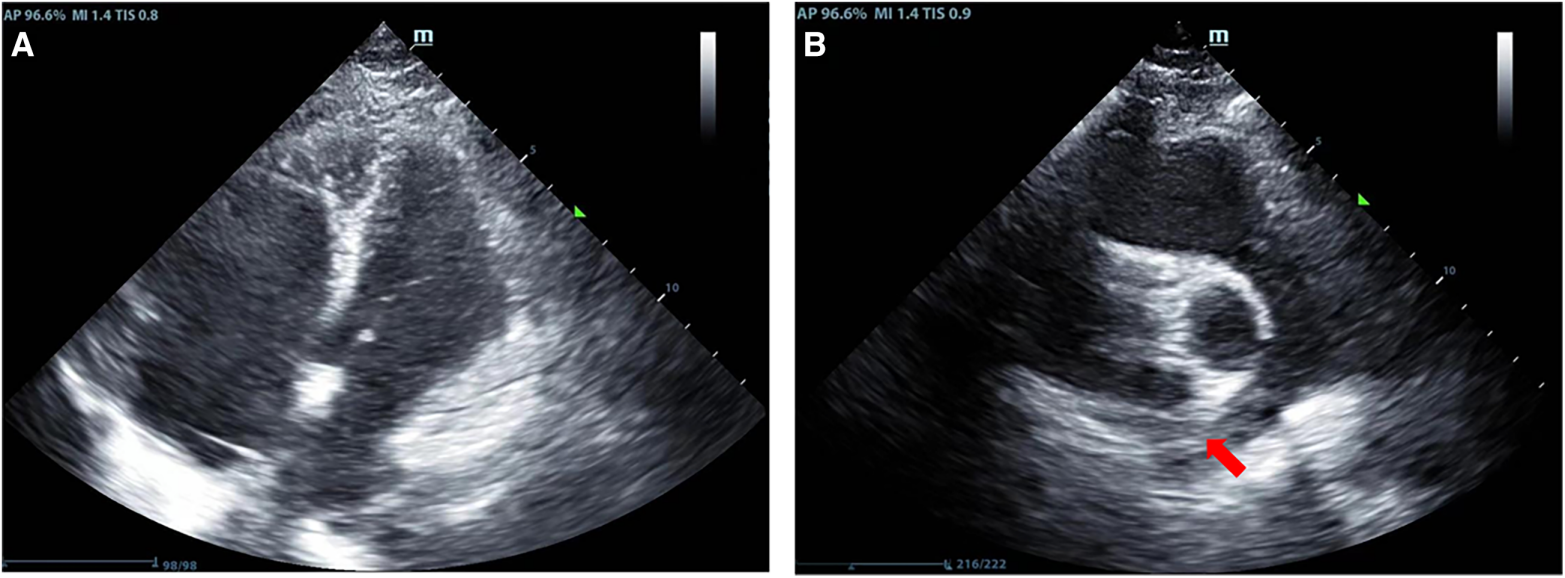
Results of the patient's echocardiography. (A) Indicates enlargement of the right heart in the patient; (B) Shows thrombus echoes in the main pulmonary artery of the right lung (red arrow).
